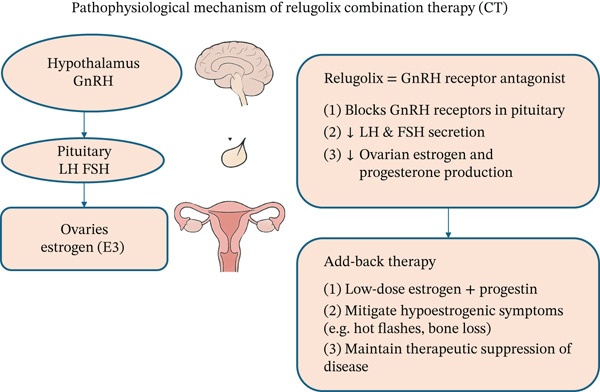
Pathophysiological mechanism of Relugolix CT.
